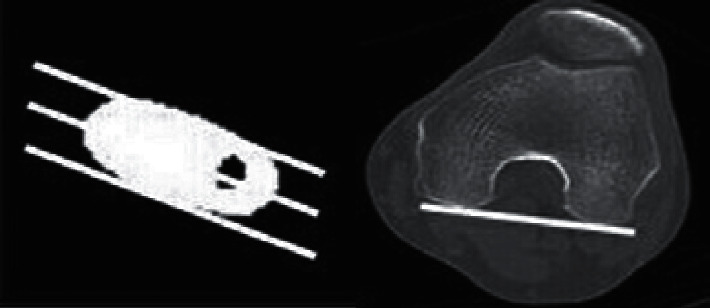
Stem rotation setting angle. The stem rotation setting angle is measured using the rotation axis of the stem from the anterior/posterior stem in the CT horizontal section with reference to the posterior condylar axis.
